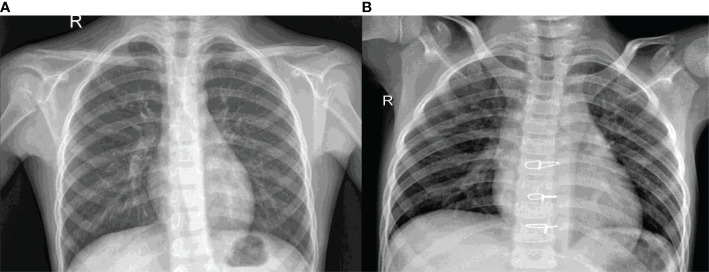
X-ray images of the lungs (A) Healthy chest X-ray (B) Pneumonic chest X-ray.
